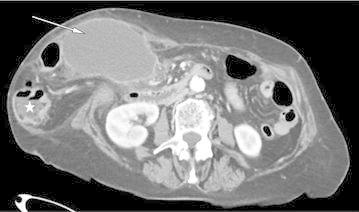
Pre-operative CT-scan. Incisional hernia containing the gallbladder (*arrow*) and digestive structure (*star*).
